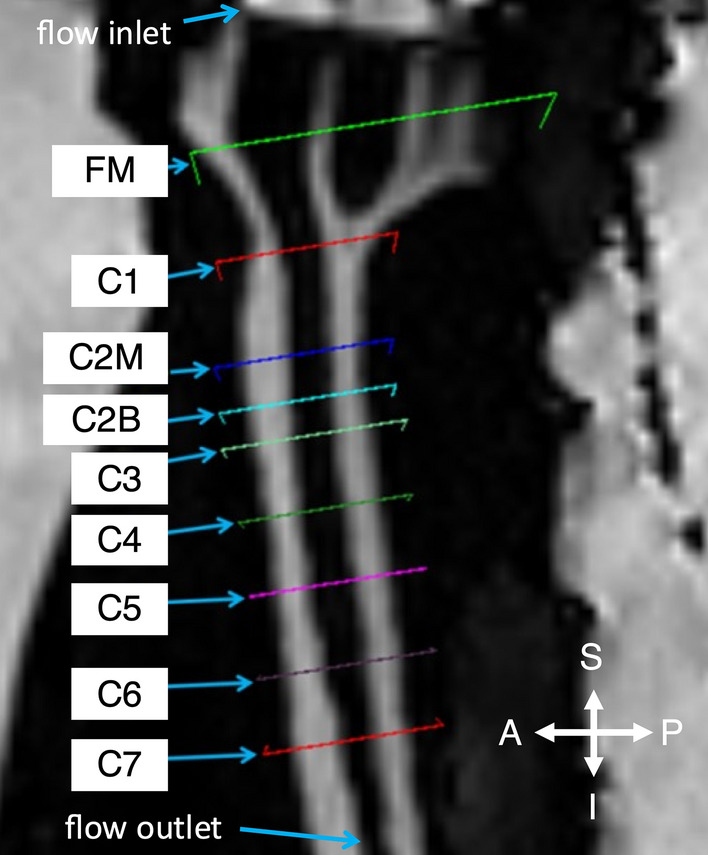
Axial positions of 2D PC MRI and 4D Flow MRI velocity measurements with flow inlets and outlets indicated. Distance between imaging planes can be found in Table 4. A = anterior, P = posterior, S = superior, I = inferior
